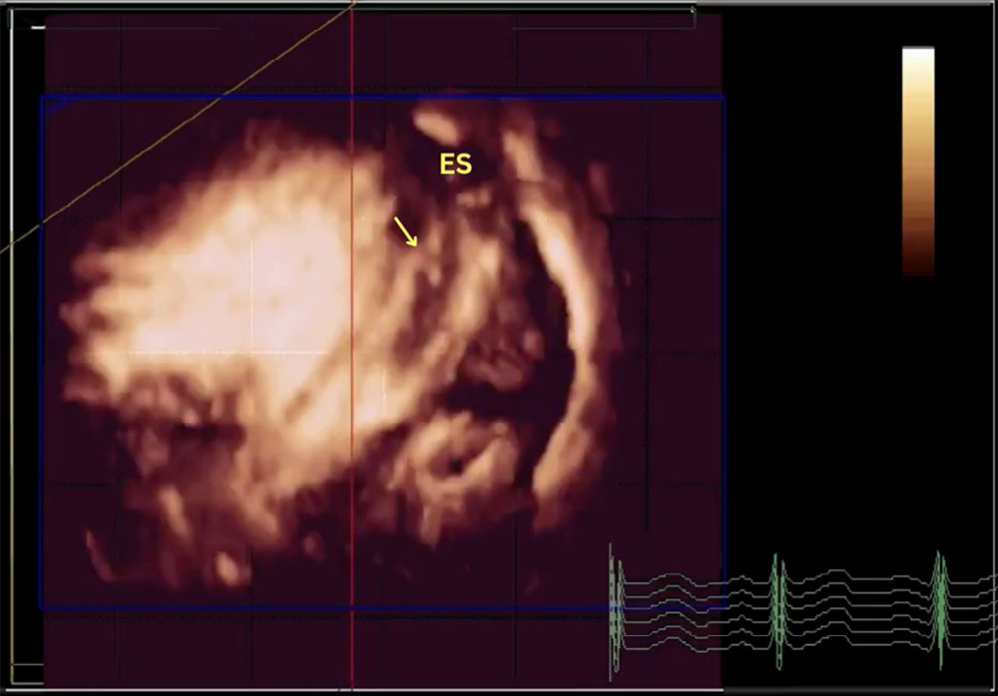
Three‐dimensional transthoracic echocardiography. Full volume mode. The arrow points to a linear structure hanging down from the ES wall. ES, esophageal stent.
